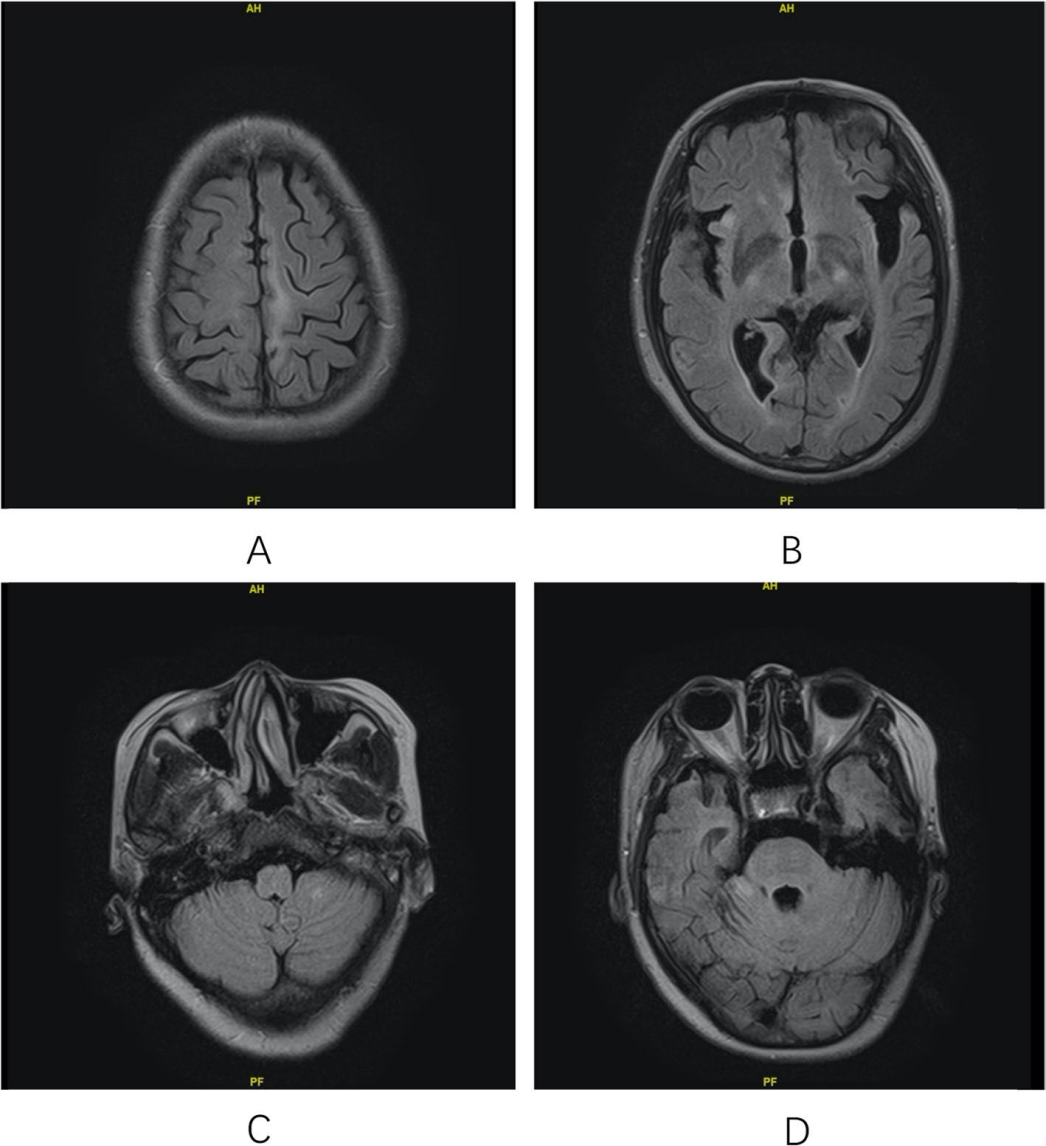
Brain MRI findings of patient No.3. The brain MRI was obtained 10 months after symptom onset, when the patient was admitted into hospital. Increased fluid-attenuated inversion recovery (FLAIR) signal could be observed in left medial frontoparietal lobe (A), right insular cortex (B), and bilateral cerebellar hemispheres (C, D). The signal abnormalities extended beyond the limbic system
